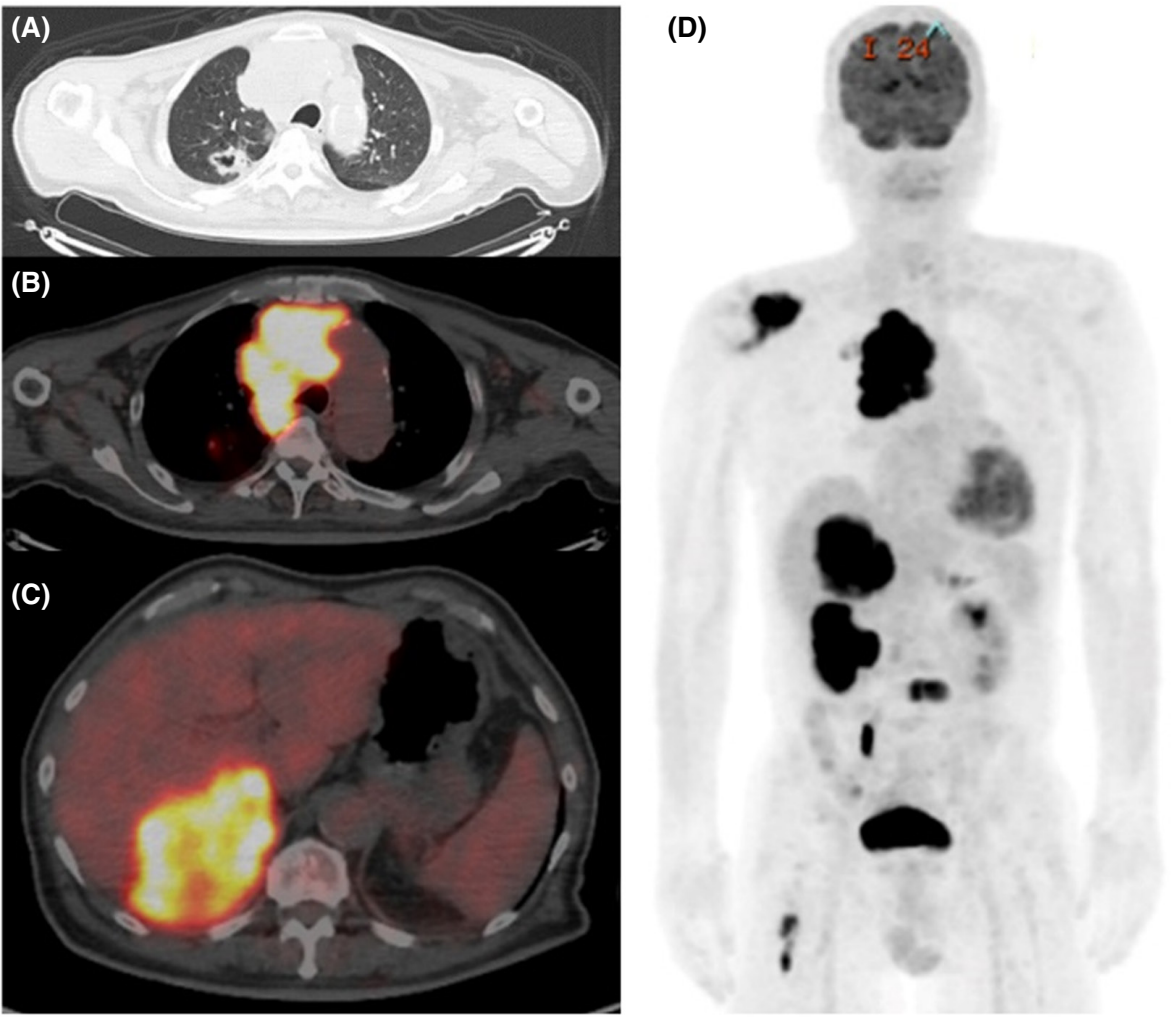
PET CT scan. (A) A 3.4 cm primary tumor in the right upper lobe; (B) mediastinal lymph node metastases; (C) large metastatic lesion in the right adrenal gland; and (D) multiple bone metastases. CT, computed tomography; PET, positron emission tomography
